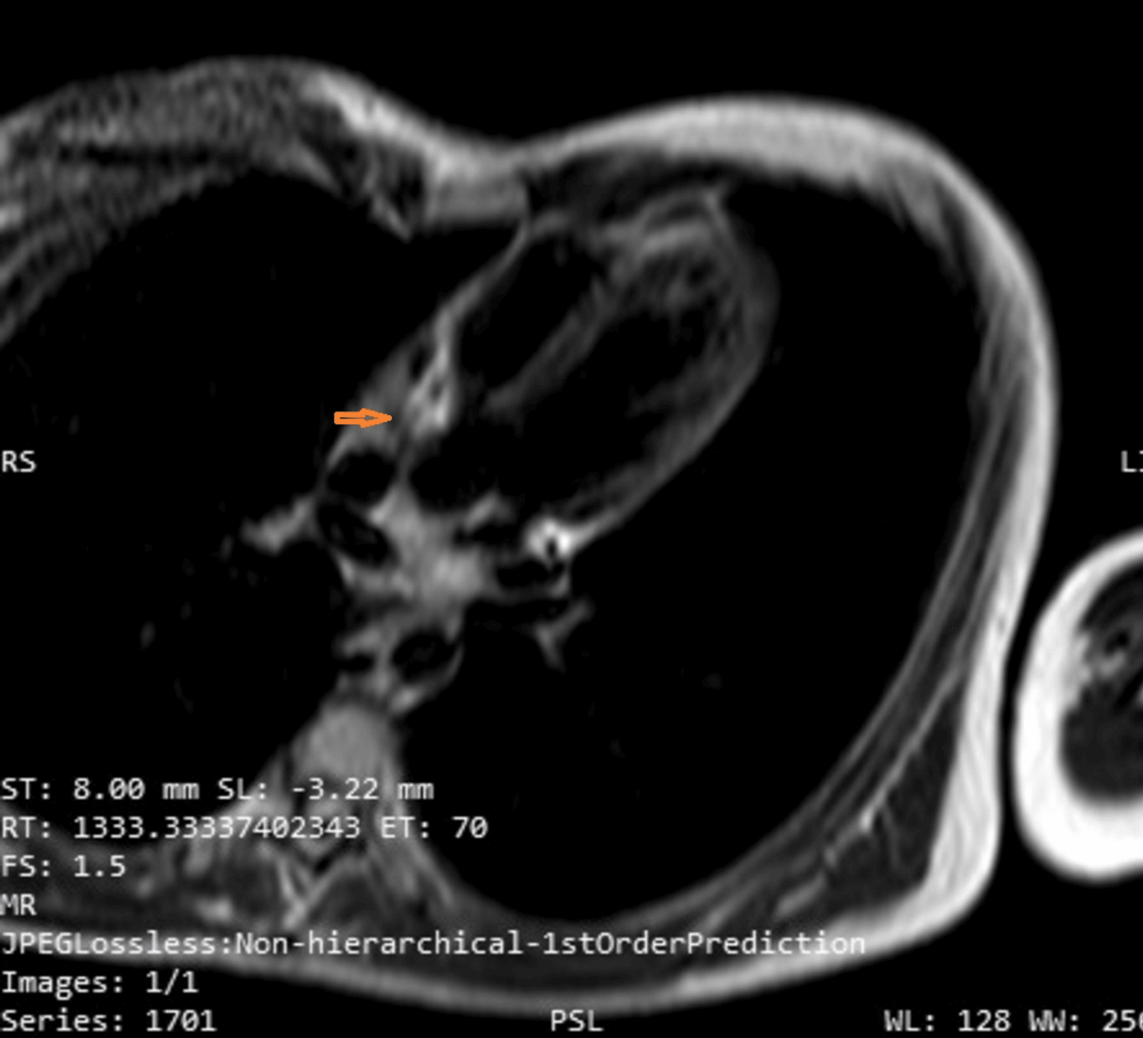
T2-weighted Turbo Spin Echo with Fat Saturation MRI sequence showing thickening of the right ventricular free wall with the muscular band extending from the tricuspid valve to the adjacent free wall (orange arrow).
